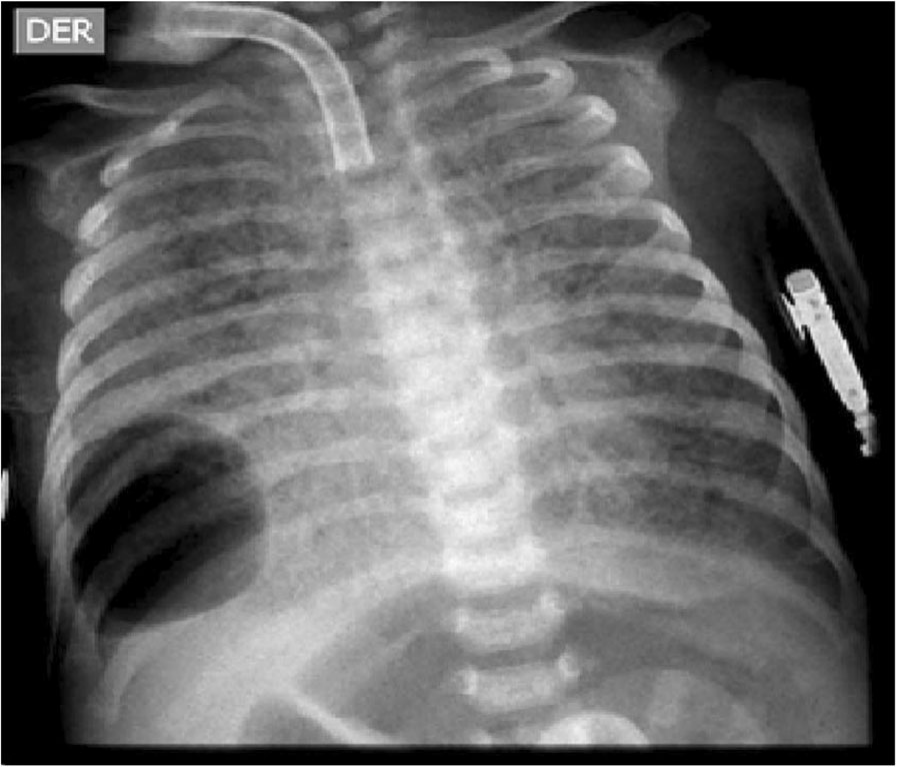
Anteroposterior chest radiograph from a newborn baby boy showing bilateral diffuse hazy granular pulmonary opacification
